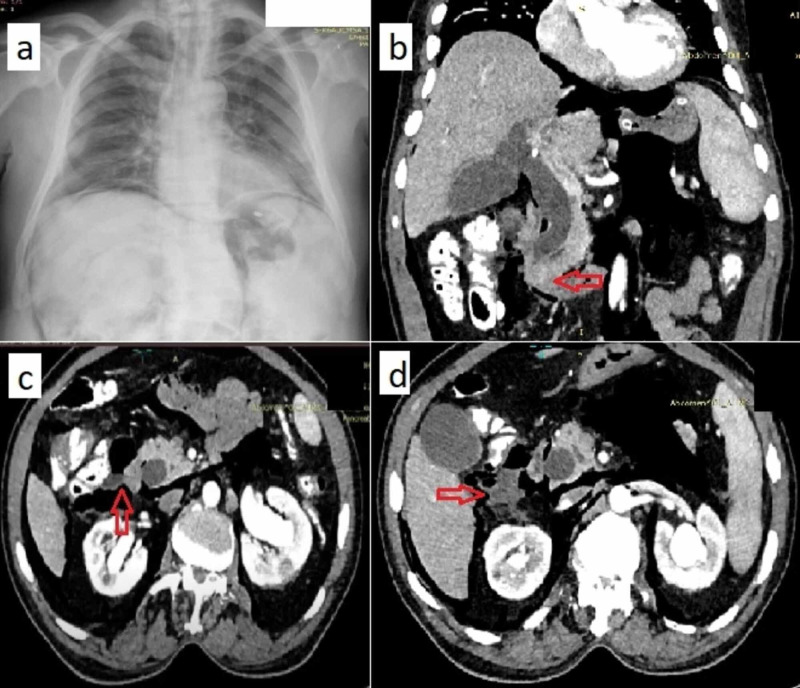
Chest radiograph and CECT Abdomen (a) Chest radiograph showing subcutaneous emphysema as well as pneumo-peritoneum and retroperitoneal free air; (b) CECT whole abdomen (coronal view) showing heterogeneously enhancing lesion in the periampullary region (red arrow) and dilated CBD; (c) and (d) CECT whole abdomen (axial views) showing perforation in the lateral wall of the duodenum with adjoining collection and pneumoperitoneum. CECT- Contrast-enhanced computed tomography
